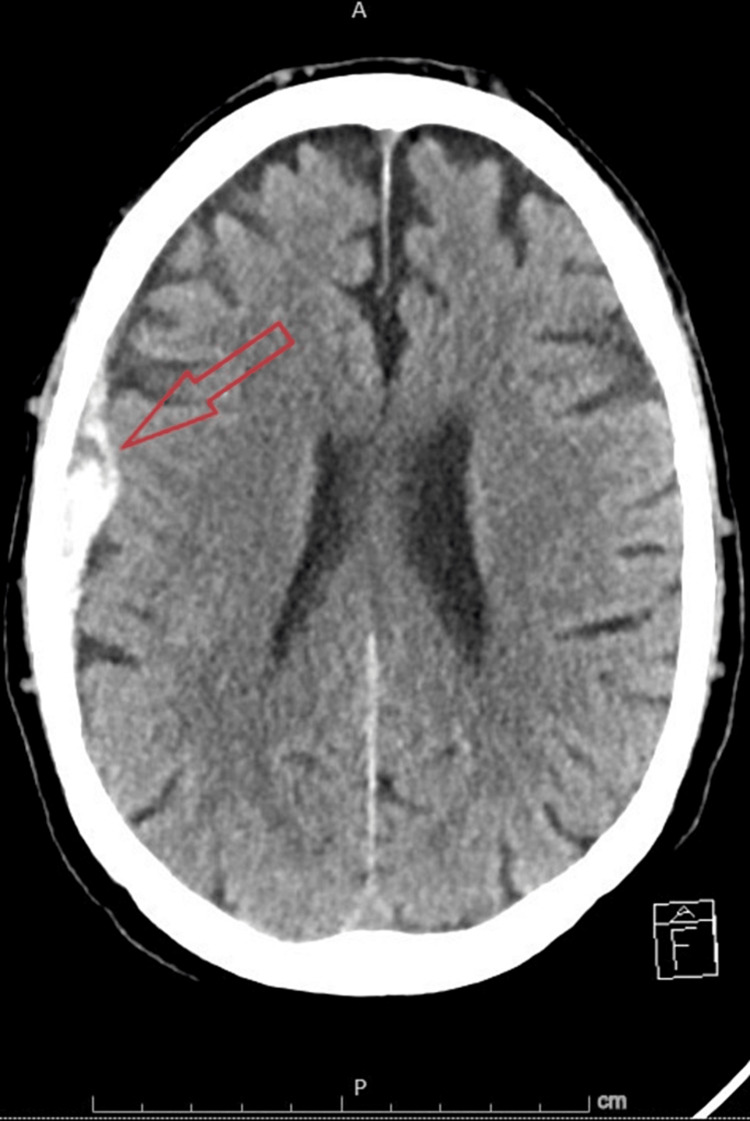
CT of the head showing acute right convexity subdural hematoma measuring up to 11 mm in maximal diameter with midline shift to the left measuring up to 2 mm.
